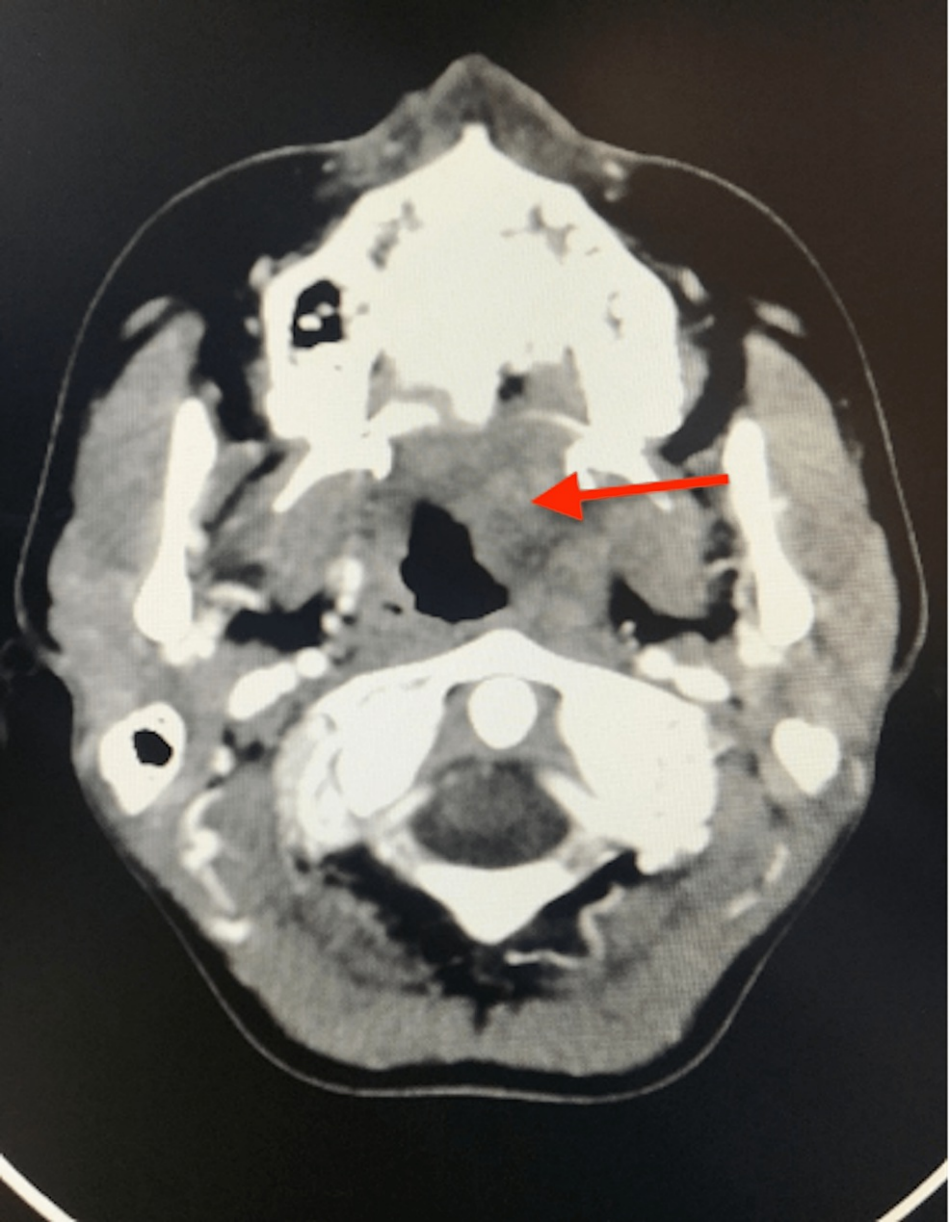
Contrast-enhanced computed tomography scan (axial section) showing a heterogeneous tissue lesion with moderately hypodense areas, well limited and responsible for a discrete mass effect on the oropharynx (red arrow). Presence of some subcentimeter bilateral jugulo-carotid nodes.
